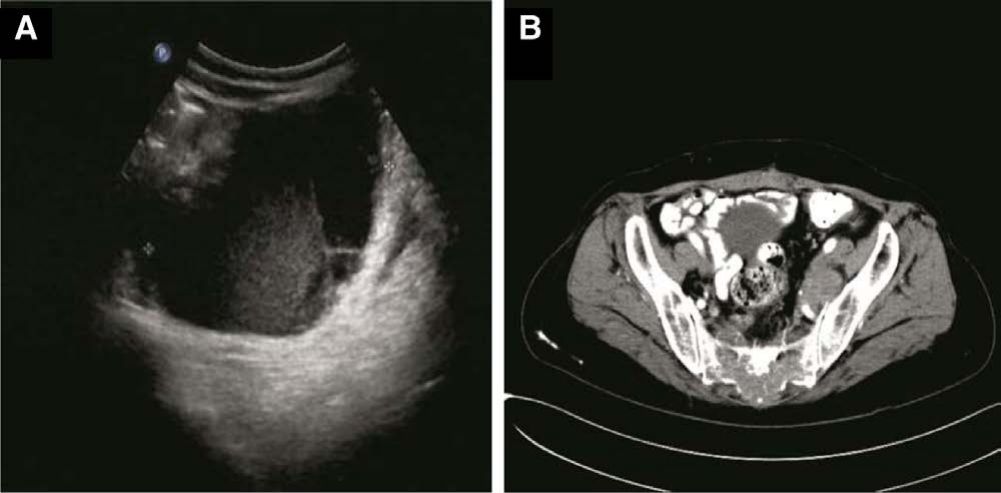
Abdominal ultrasound (A) and computed tomographic scan (B) revealed pelvic masses with a diameter of 8 cm × 6 cm × 4 cm tumor in left pelvic and another one of 6 cm × 4 cm × 3 cm closely relative to posterior wall.
